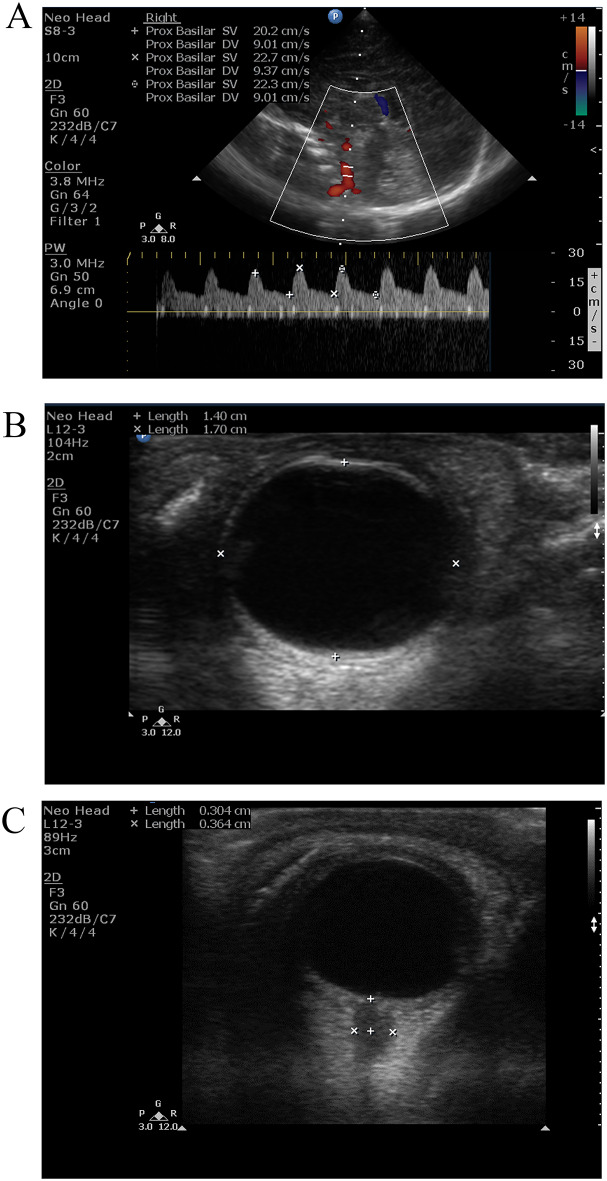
RI of BA in patient with severe HIE within first 12 hours after birth, with low RI indicating altered cerebral perfusion in HIE and a reduction in cerebrovascular resistance (A). ocular sonography showed ocular dimensions (B) and large optic nerve sheath diameter (C)
